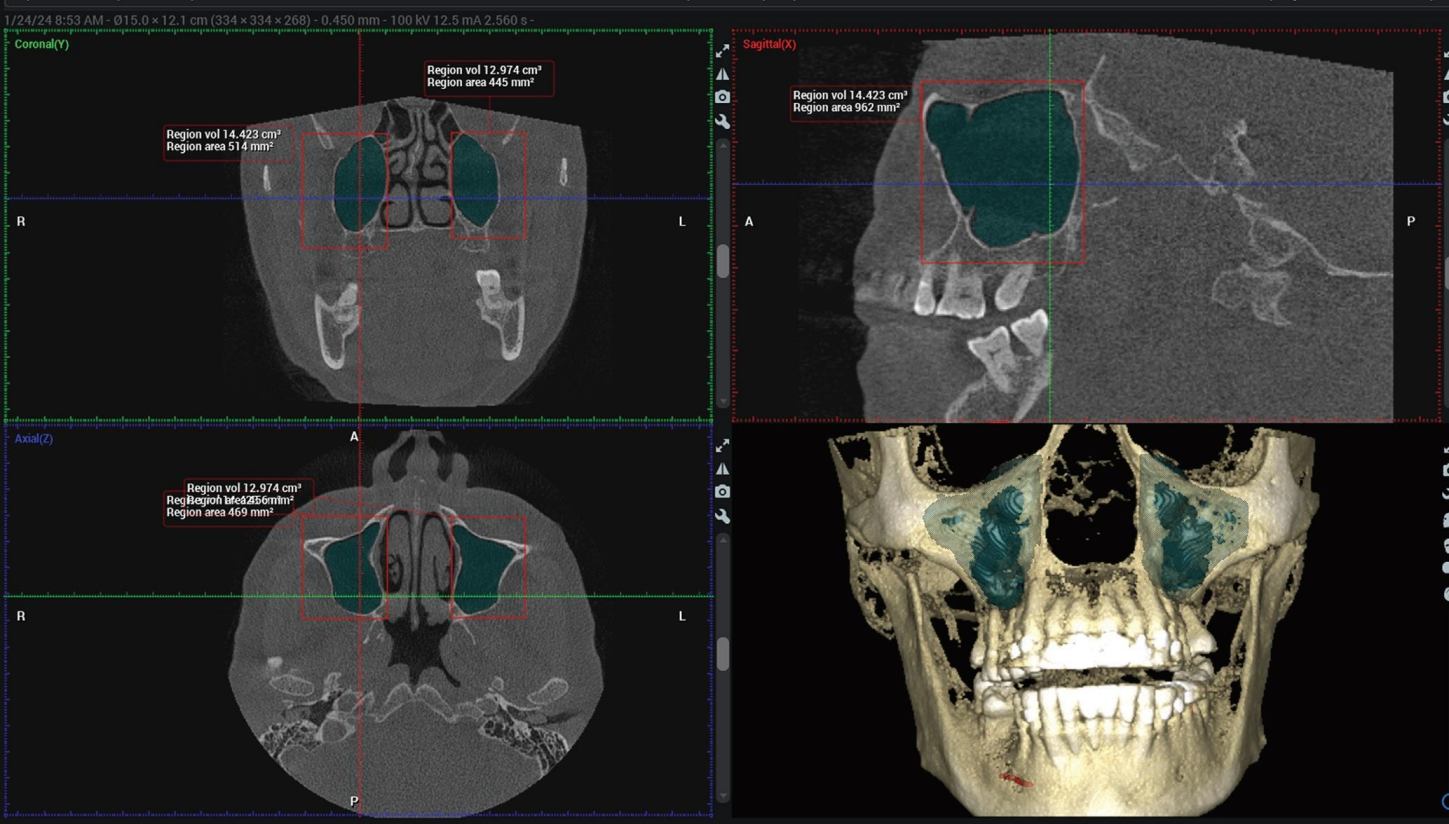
Multiplanar CBCT views in coronal, sagittal, and axial planes, along with a 3D reconstruction, demonstrating bilateral segmentation of the maxillary sinuses highlighted in green using the region‐growing tool.
